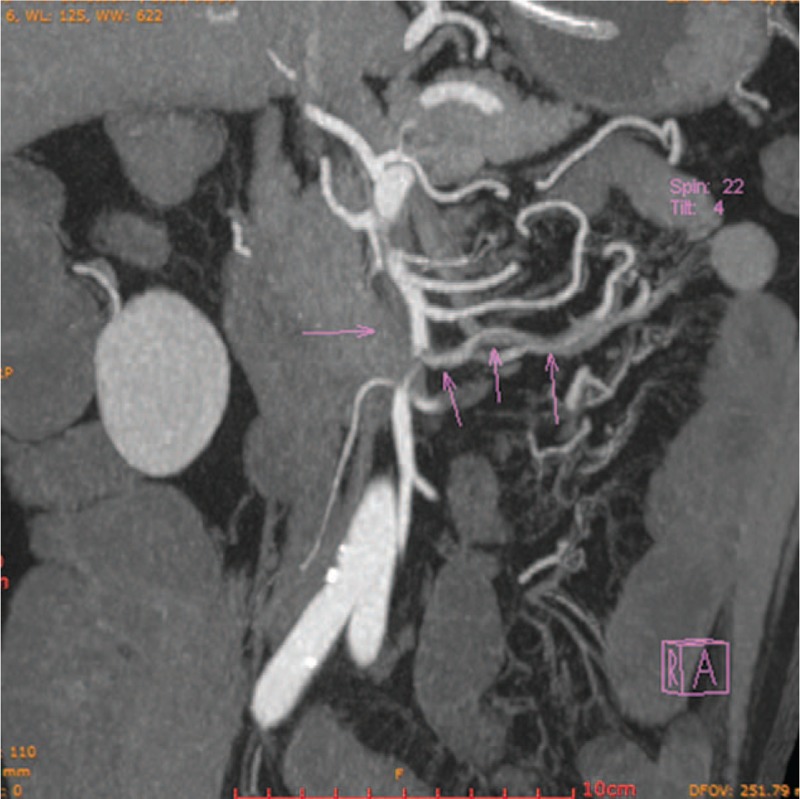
Computed tomography scan of the abdomen showing the left 2 branches of the superior mesenteric artery were appeared “low density filling defect sign,” and the true lumen were extremely constrictive (arrow).
